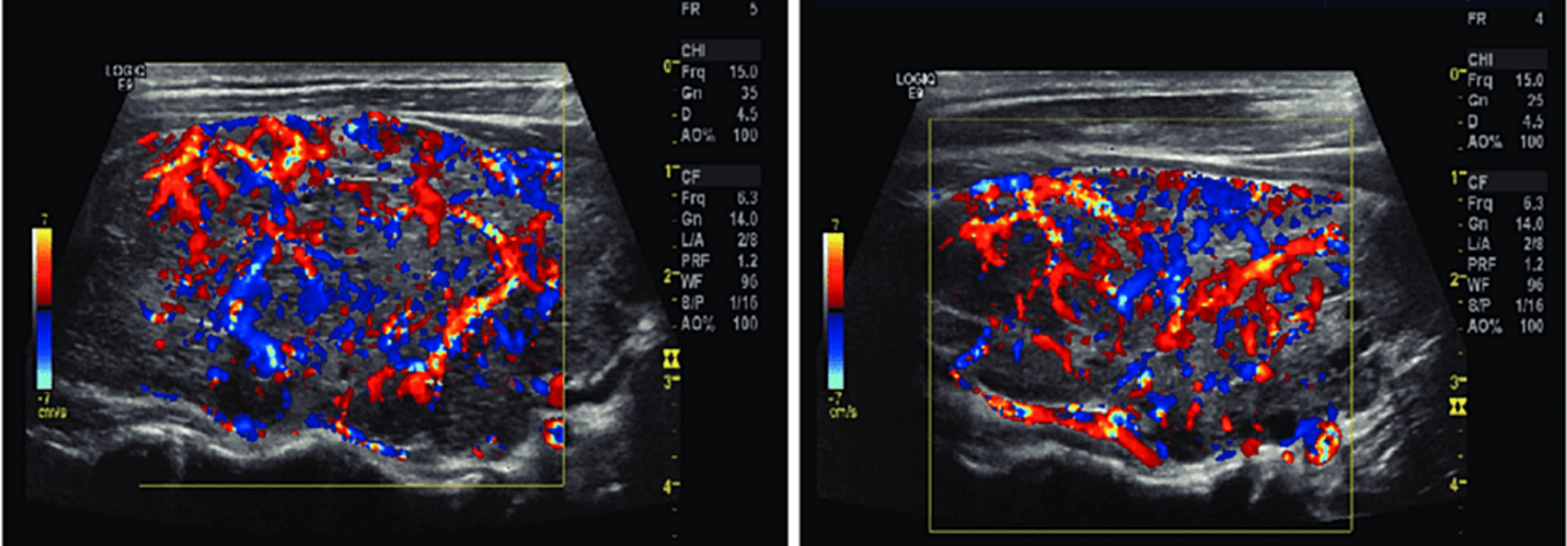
Doppler Ultrasonography Longitudinal Views of the Thyroid Gland Showing Diffuse Enlargement and Increased Vascularity
